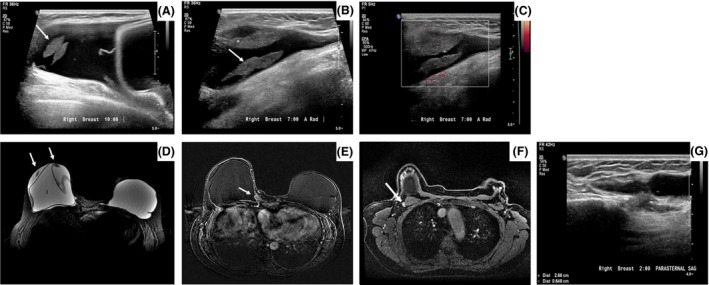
Breast implant‐associated anaplastic large‐cell lymphoma. Radiology. Ultrasound demonstrates a large fluid collection which contains thick septations and solid detritus (arrows) adjacent to the saline implant (A, B), with no evidence of vascularity on Power Doppler imaging (C). Axial MRI STIR sequence obtained in the prone position demonstrates the peri‐implant fluid collection with solid detritus (arrows) surrounding the implant (I) (D). Postcontrast subtraction axial sequence demonstrates a 2.3 cm irregular enhancing mass along the medial aspect of the right implant (arrow) (E). T1 Fat Sat with contrast demonstrates a prominent level 1 axillary lymph node without fatty hilum (F). Second look ultrasound confirmed the presence of the mass that was identified deep to the implant in the supine position (G).
